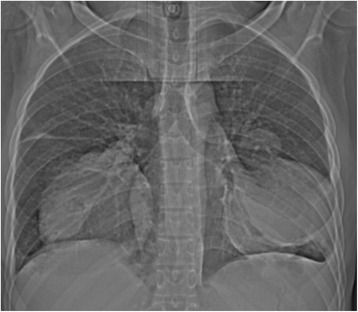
Chest X-ray (posteroanterior view) displaying bilateral, ovoid-shaped perihilar opacities extending to lower lung zones with loss of silhouette sign on the left cardiac border
